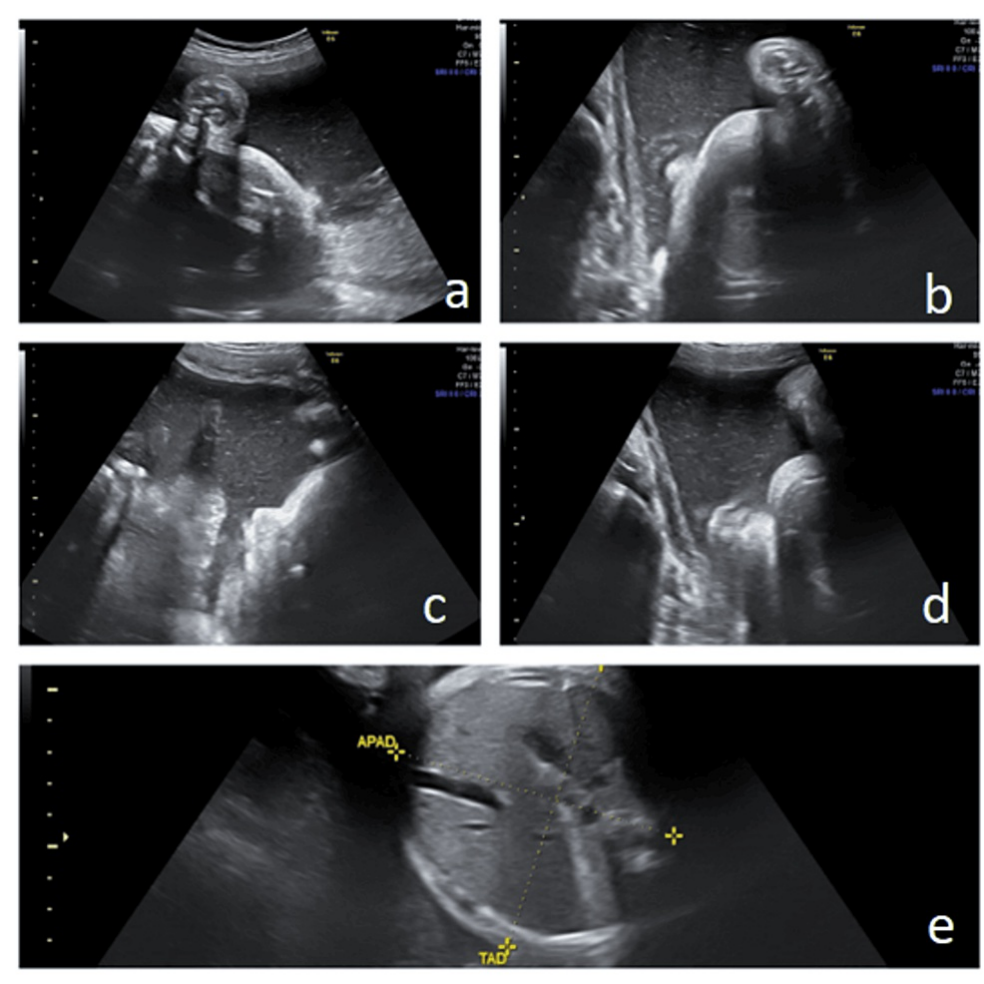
Ultrasound scan at 35 weeks demonstrates increased amniotic fluid index. The dimensions of abdomen are normal and the stomach is visible. During the scan, normal swallowing movements of the fetus were detected. a) First quadrant, b) second quadrant, c) third quadrant, d) fourth quadrant, e) fetal abdomen with normal size for the gestational age
